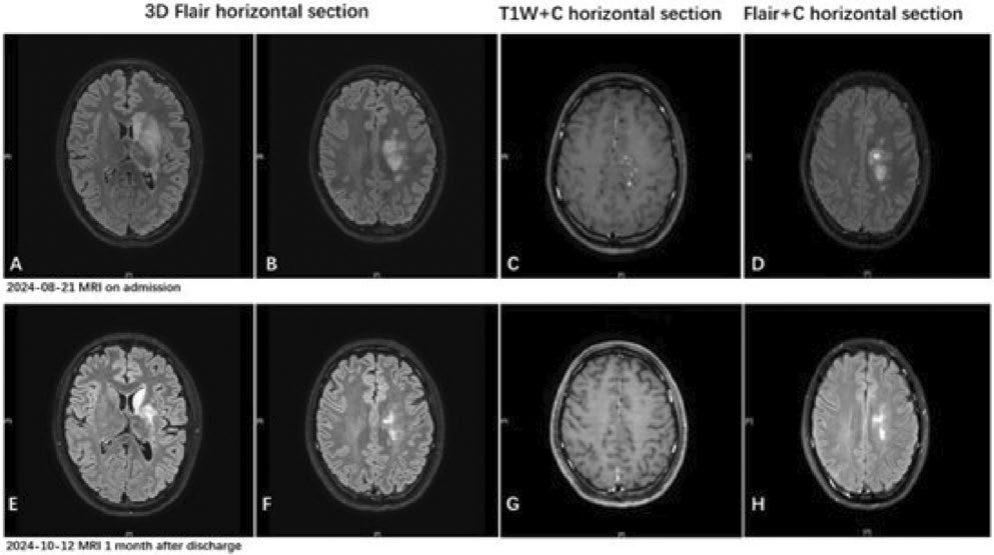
FIGURE 1 The MRI at admission, the review MRI during hospital stay, and the review MRI at one month post‐discharge.
